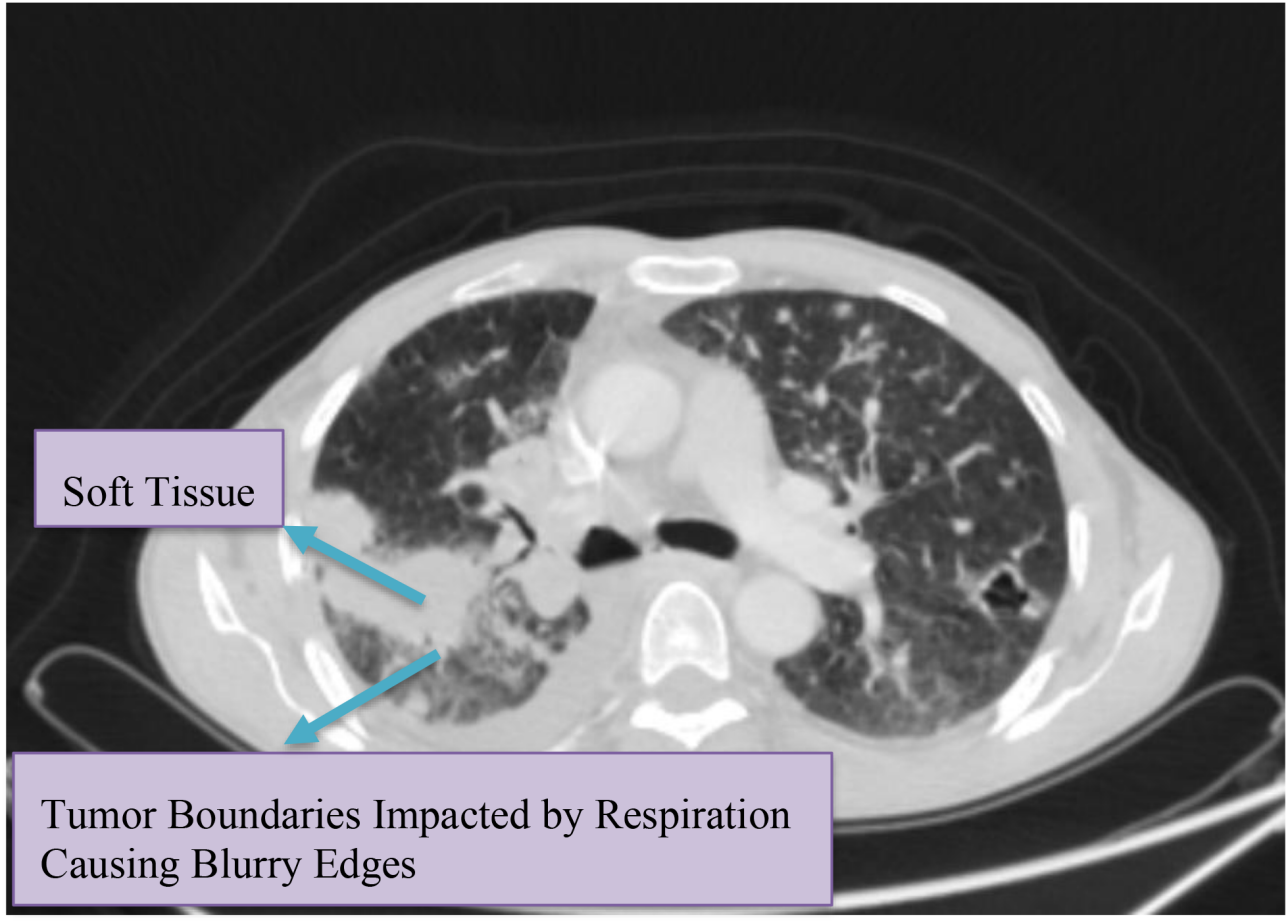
HU sampling across tumor volumes and boundaries over multiple CT slices to capture respiration-related density variations.
